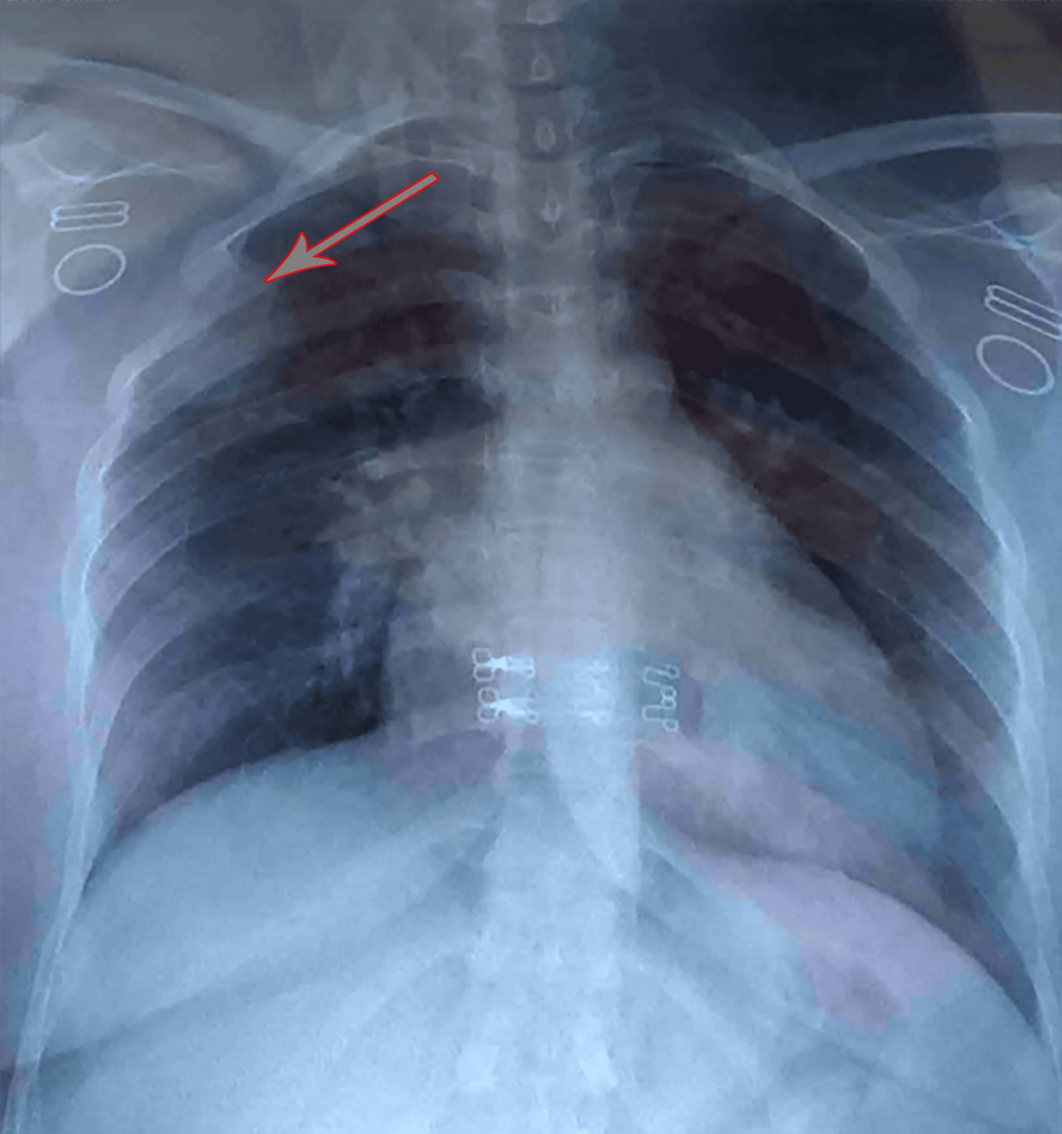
Chest X-ray shows the lung hydatid cyst (arrow).
